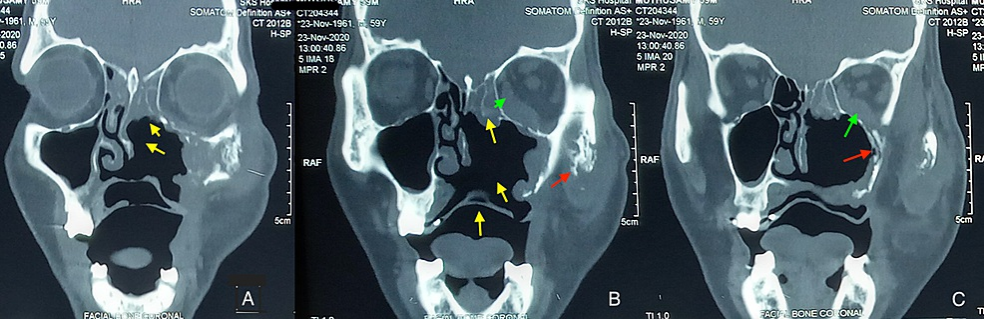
Post sequestrectomy MDCT coronal section of facial bones (A-C) reveals changes showing osseous defects in the hard palate, left ethmoidal sinus, medial wall and floor of left maxillary sinus (yellow arrow), ill-defined bony erosion involving the lateral wall of maxillary sinus, left zygomatico-maxillary process (red arrow), and inflammation of inferior and medial rectus (green arrow). MDCT: multidetector CT scan
